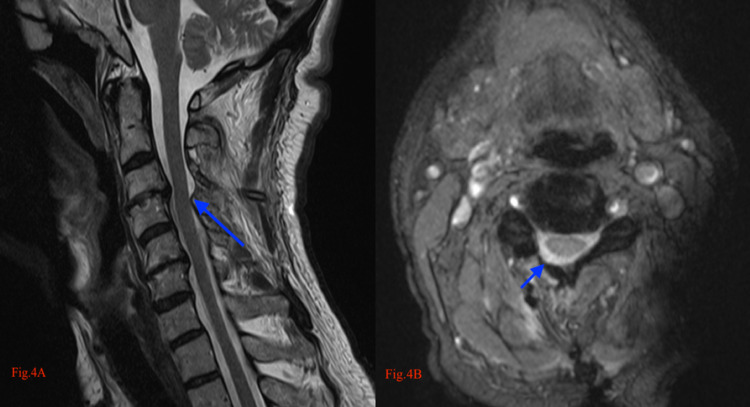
(A) Postoperative MRI T2-sagittal and (B) T2-axial sequences; blue arrows show the region after surgery (ULBD) ULBD: unilateral approach and bilateral decompression
